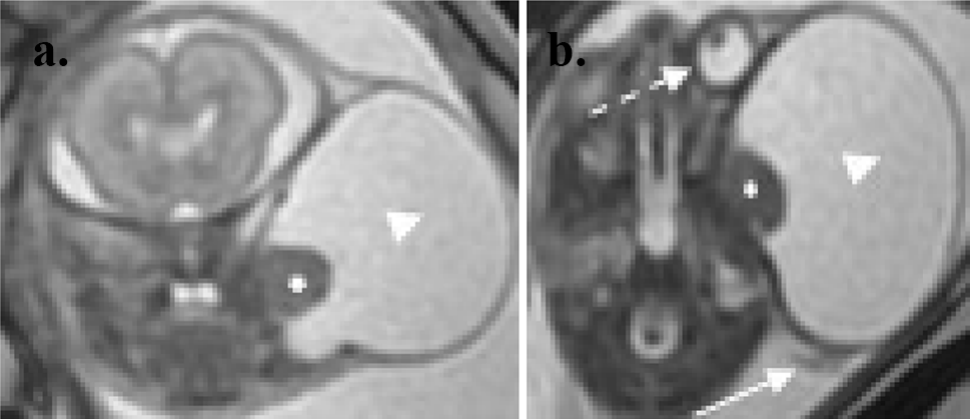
MRI at 23 weeks gestation. Coronal a and axial b T2-weighted MRI of the fetal head and neck demonstrate a large cystic lesion (arrowhead) in the left infratemporal region with a smaller solid component in its medial aspect. It is causing significant distortion of surrounding structures including displacement of the left orbit (dashed arrow) and ear (arrow)
